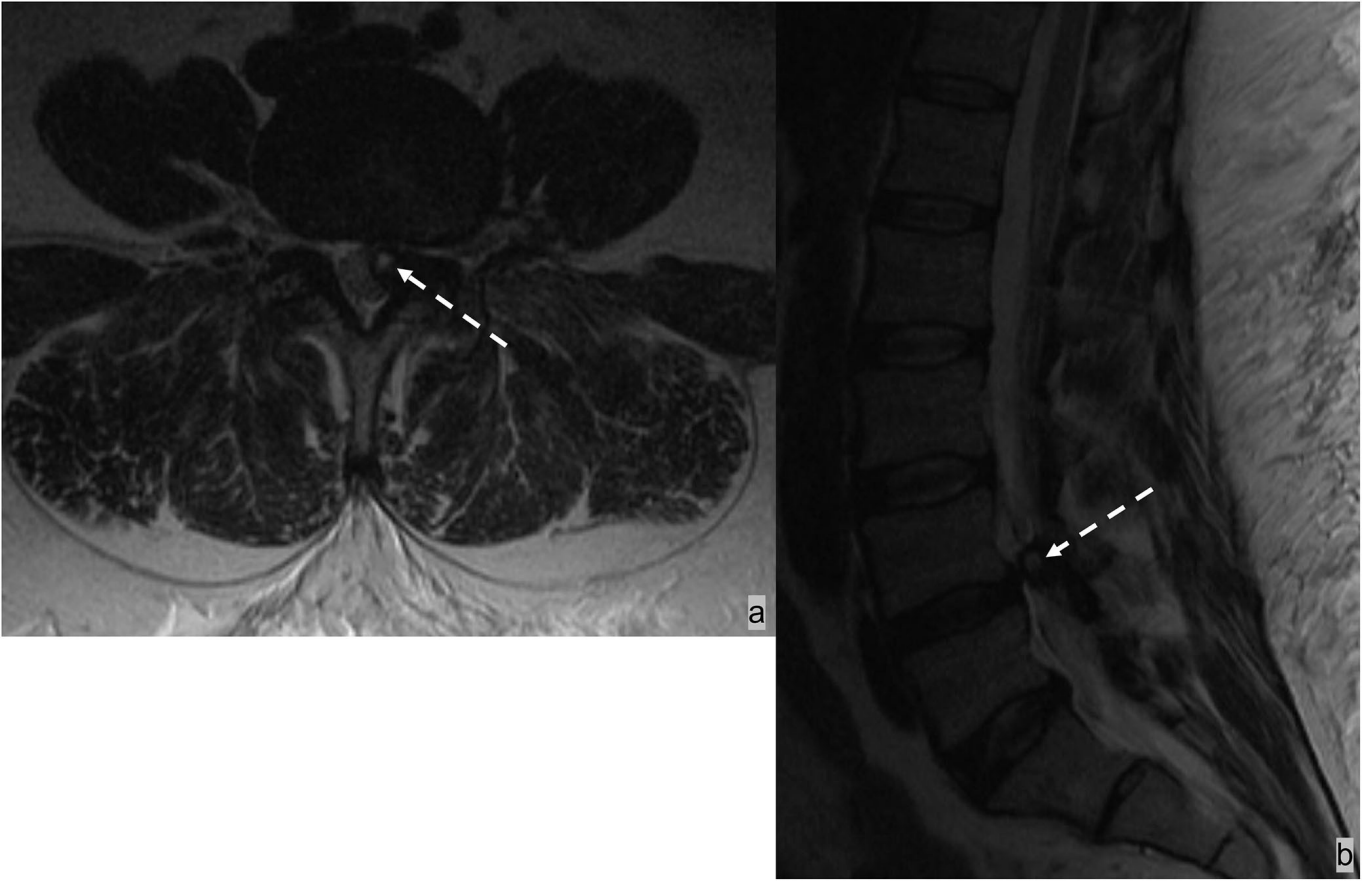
Patient presented with back pain radiating to the left lower extremity. MRI demonstrated a T2 hyperintense synovial cyst in the epidural space abutting the left facet joint at L4-5, which resulted in stenosis of the left subarticular recess and impingement of left L5 traversing nerve root.
